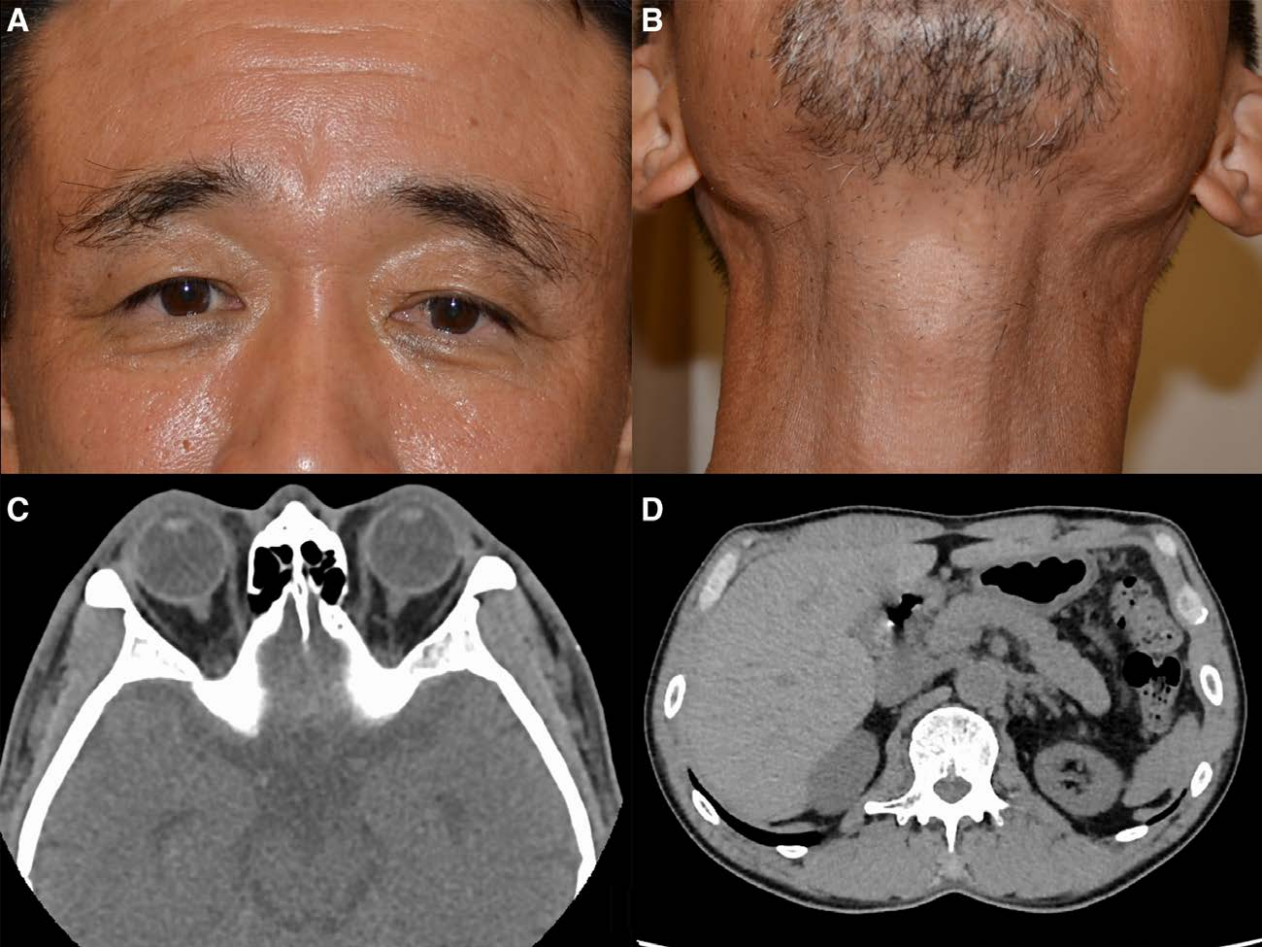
Clinical findings at 4-year follow-up period. (A, B). Photos of the patient. Swelling of the upper eyelids (A) and submandibular regions (B) subside. (C). An axial orbital CT showing no enlargement of the lacrimal gland on either side. (D). An axial abdominal CT showing improvement of the enlarged pancreas and thickened abdominal aortic wall. CT = computed tomographic.
